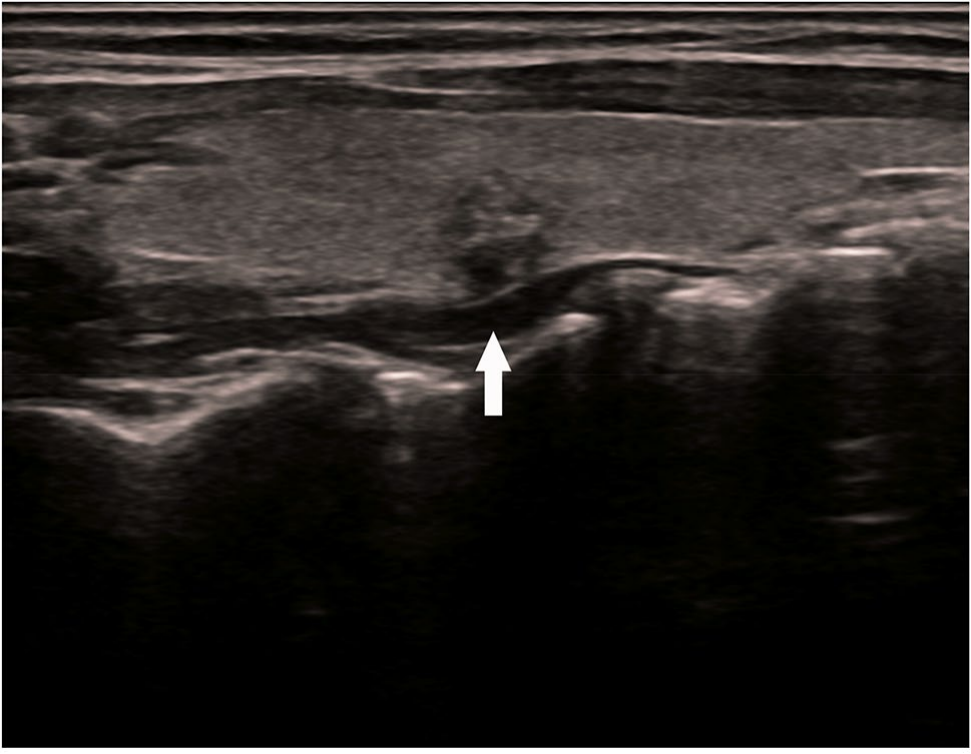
An ultrasound image of a 41-year-old female patient with papillary thyroid carcinoma.
